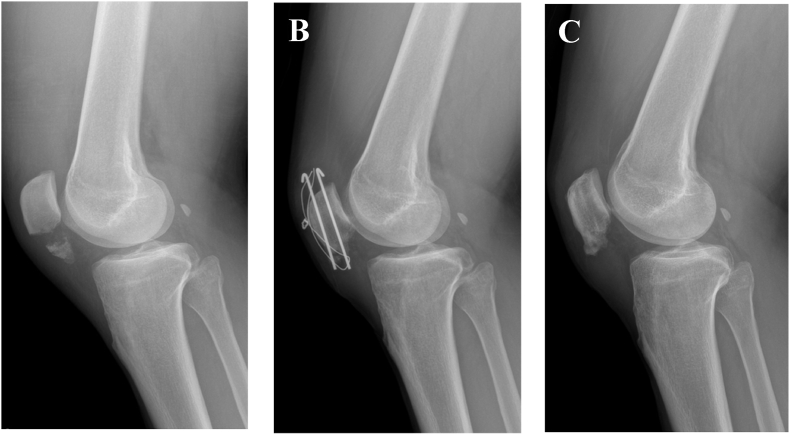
A 50-year-old man underwent tension band wiring for a left patellar inferior pole fracture. (A) Preoperative knee radiograph (B) Immediate postoperative knee radiograph (C) 24 months postoperatively.
